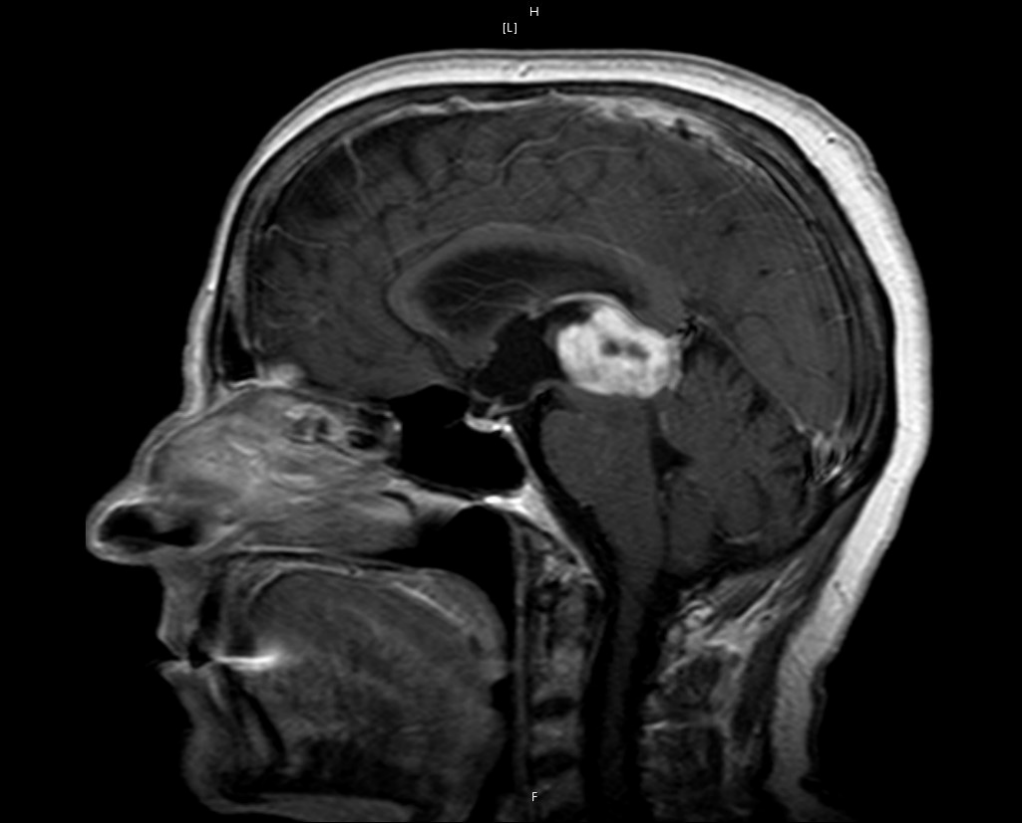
Preoperative MRI Sagittal MRI with contrast revealing an enhancing pineal region lesion suspicious for a glial-type lesion.
